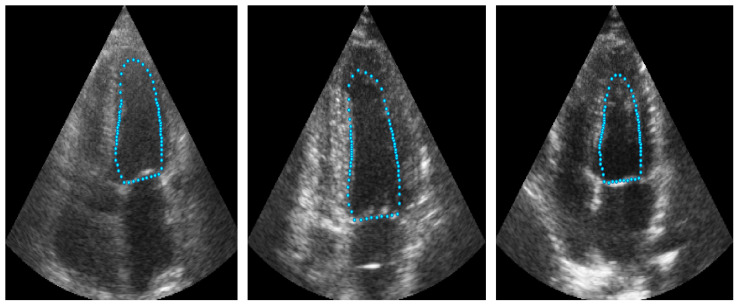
Example of landmarks sampled in LV ultrasound images.
